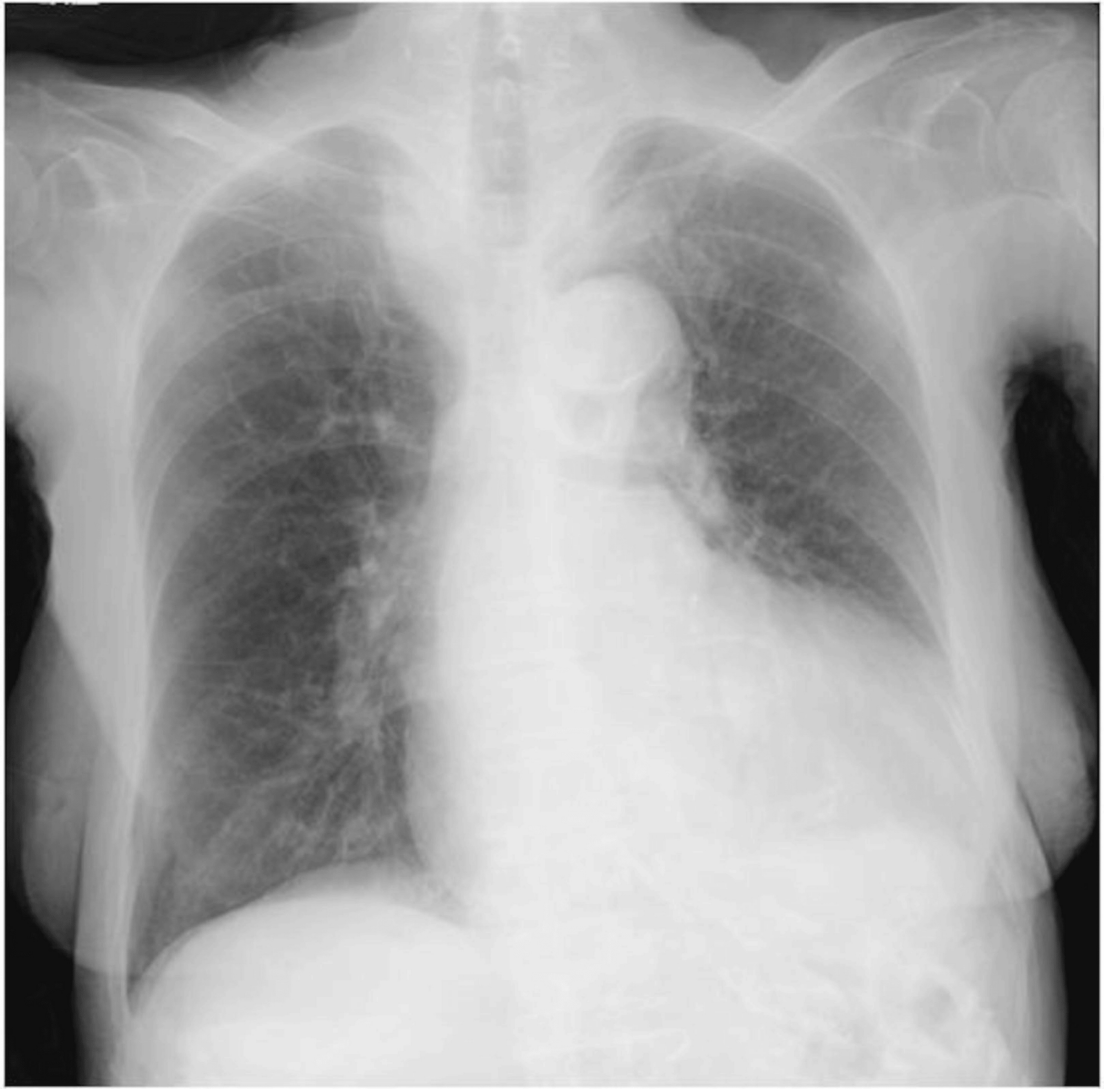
Chest radiograph in case 1. Chest radiography revealed cardiomegaly.
